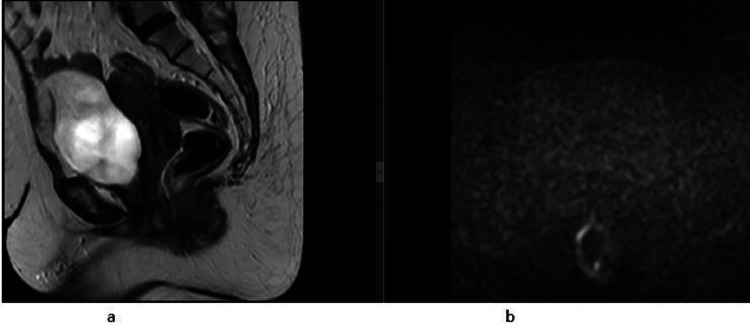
MRI pelvis performed post-chemo-radiation There is interval resolution of previously noted abnormal signal intensity in the upper third of the vagina, the base of the urinary bladder appears grossly unremarkable, and no abnormal diffusion restriction was noted. The pelvic side wall is clear, suggesting a complete response to therapy.
